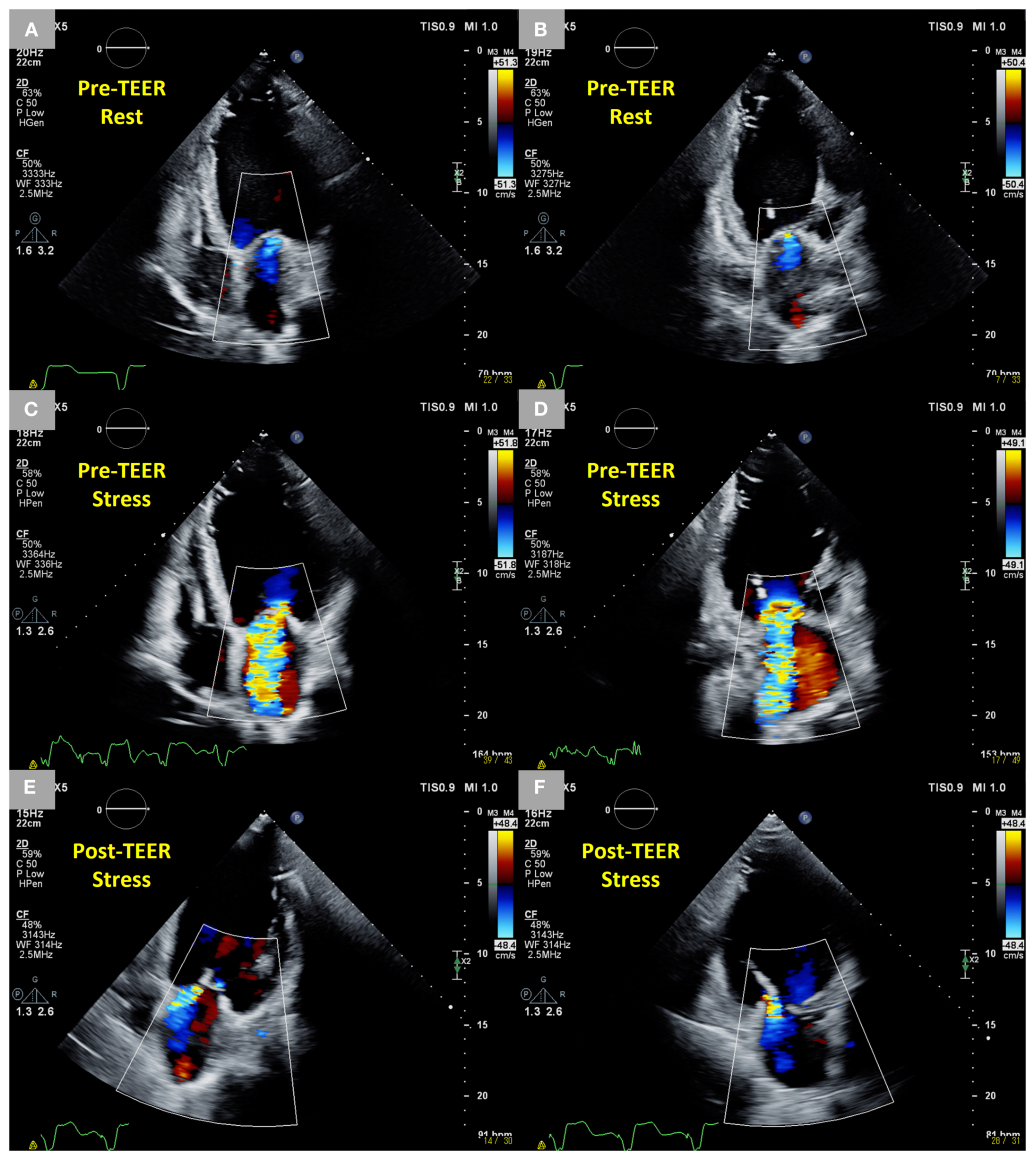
Dynamic changes in SMR during exercise stress echocardiography before TEER and controlled SMR following TEER during exercise stress echocardiography in an 83-year-old male patient who had an anterior and inferior old myocardial infarction and heart failure with reduced ejection fraction after CRT. (A,B) Trivial SMR at rest in two-dimensional color Doppler images from four-chamber and two-chamber views. (C,D) Dynamic severe SMR (EROA 0.52 cm^2^) under stress in two-dimensional color Doppler images from four-chamber and two-chamber views. (E,F) Mild MR under stress 6 months after the TEER in two-dimensional color Doppler images from four-chamber and two-chamber views. SMR, secondary mitral regurgitation; TEER, transcatheter edge-to-edge repair; CRT, cardiac resynchronization therapy; EROA, effective regurgitant orifice area; MR, mitral regurgitation.
